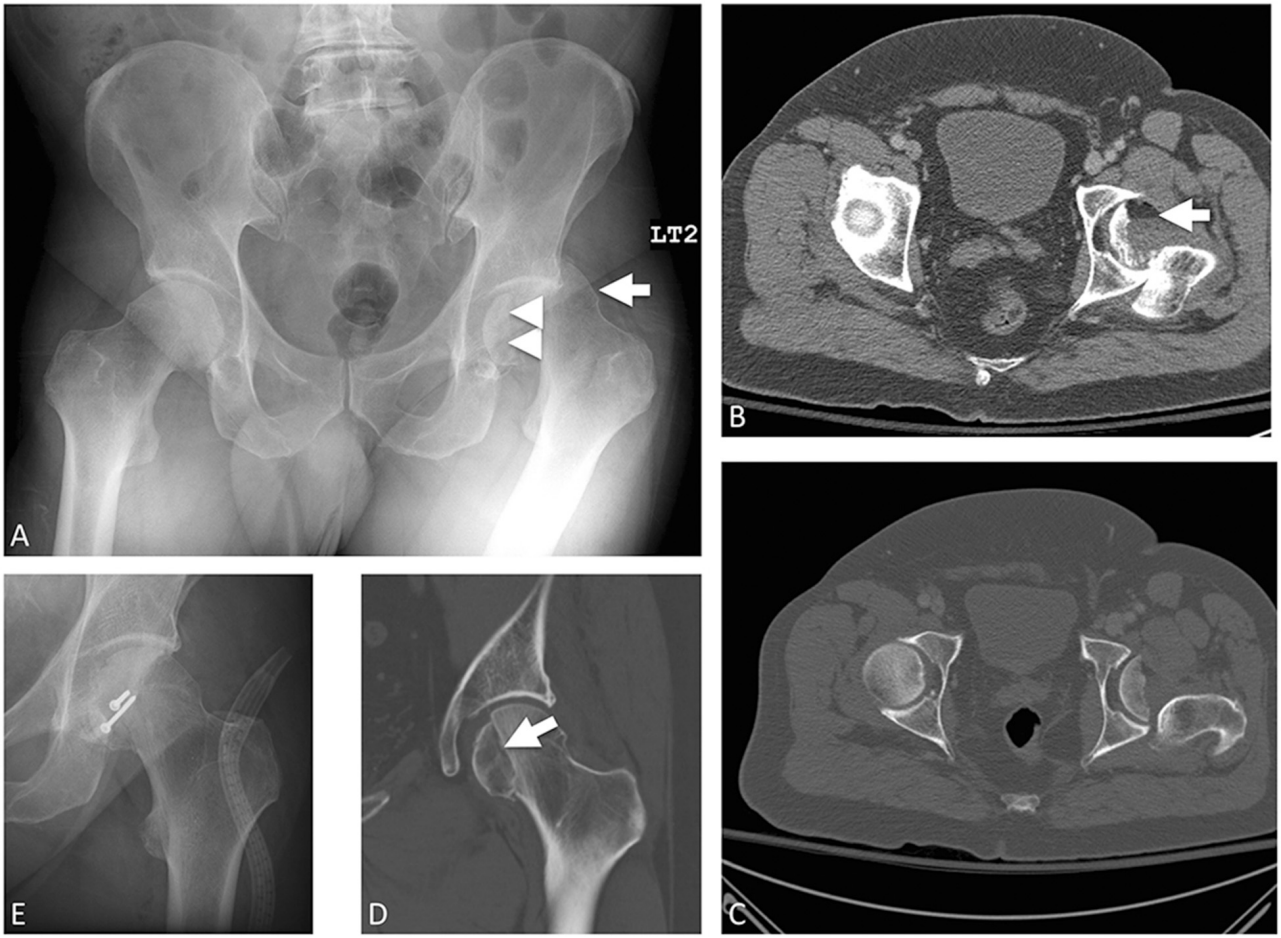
Entire treatment course of a Pipkin type IV femoral head fracture in a patient with posterior hip dislocation status post motor vehicle collision. Initial AP pelvic radiograph (a) with posterior superior femoral head dislocation (*arrow*) and femoral head fracture, with a residual femoral head fragment remaining in the acetabulum (*arrowhead*). b Axial CT showing lipohemarthrosis, with floating fatty liquid suspending atop pooling blood (*arrow*), resulting from traumatic exposure of the marrow. c Bone windows from axial CT. Femoral head fracture and posterior dislocation better demonstrated. d Post reduction hip CT coronal. Minimally displaced fracture line visible (*arrow*). e Post-operative AP hip radiograph with femoral head screws
